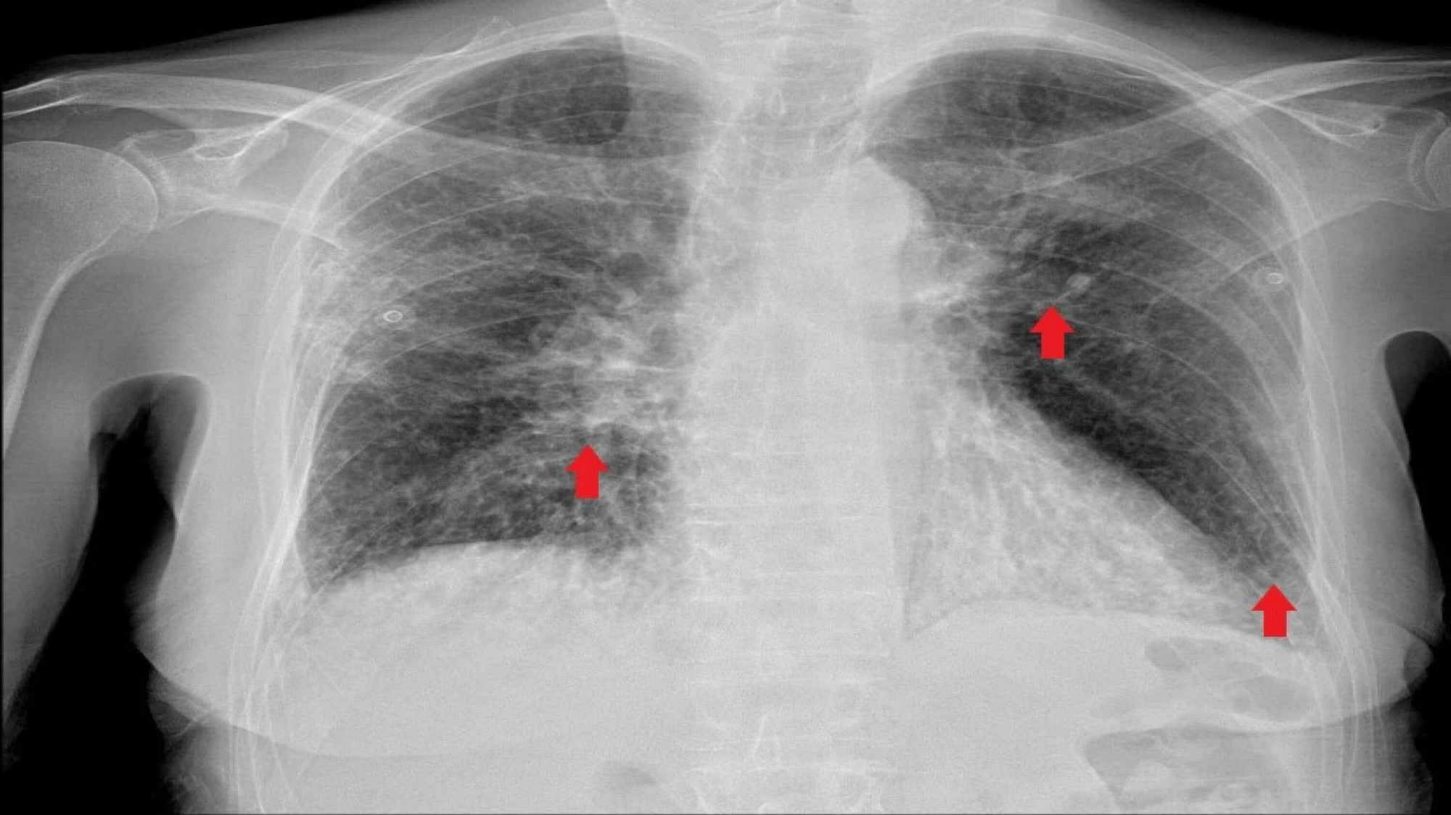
Chest X-ray showing multifocal infiltrates.
